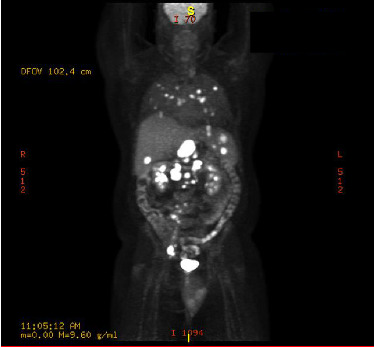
PET/CT two months prior to CAR-T therapy.
